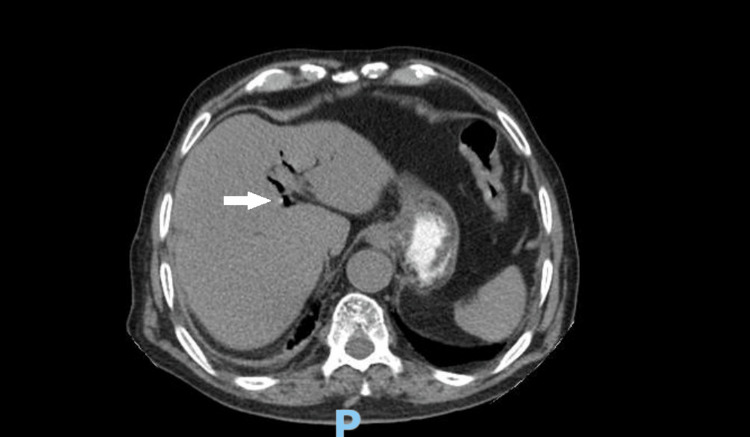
CT abdomen showing pneumobilia The arrow points to the air in the intrahepatic biliary tree.
